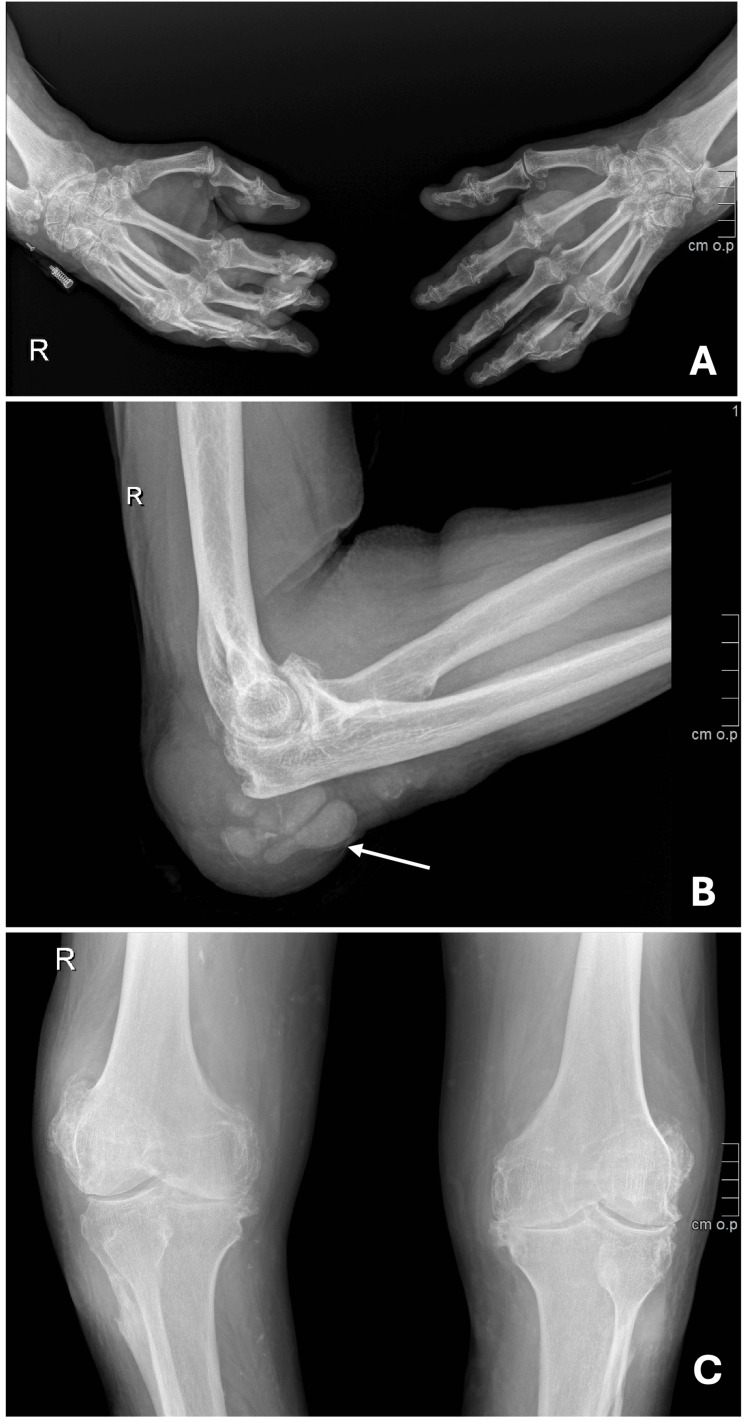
Radiographic features of tophaceous gout (A) Hand radiograph showing multiple erosions and prominent osteophytes. (B) Right elbow radiograph demonstrating punched-out periarticular erosions with overhanging edges and increased periarticular soft-tissue density compatible with tophi (arrow). (C) Bilateral knee radiographs showing severe knee osteoarthritis with large hook-like osteophytes and erosions of the fibular head.
